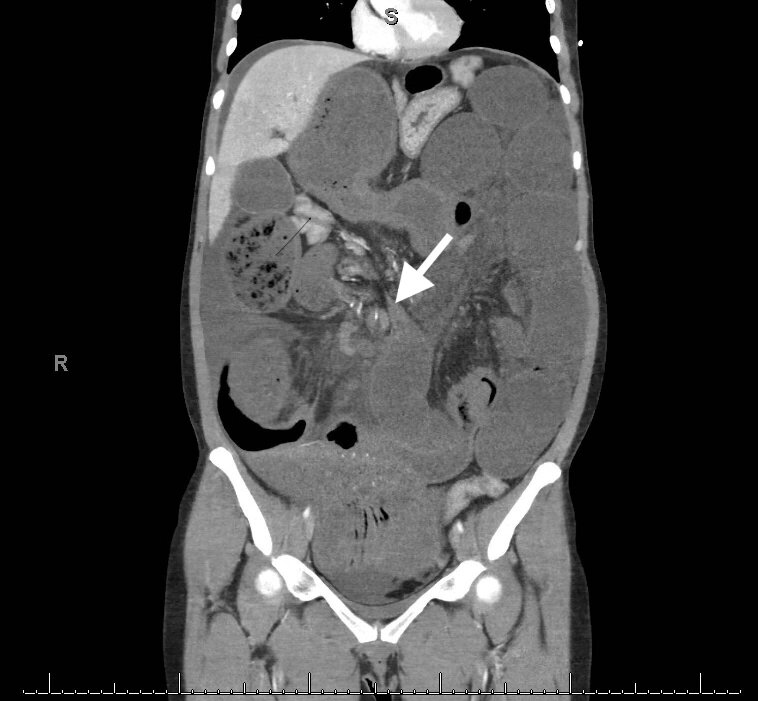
Coronal view of the patient’s computed tomography, showing one of the two transition points (arrow) noted of the high-grade small bowel obstruction located in the right mid-abdomen. There was a small amount of free fluid in the dependent aspects of the abdomen, likely reactive.
